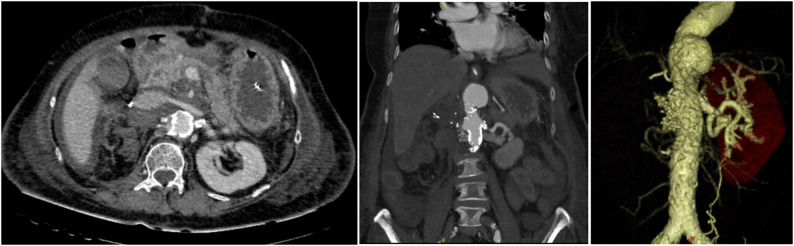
Post-operative computed tomography does not show any leakage around the patch closure. Small granular hyperdensities around the patch should be surgical pledgets and hemostatic material
